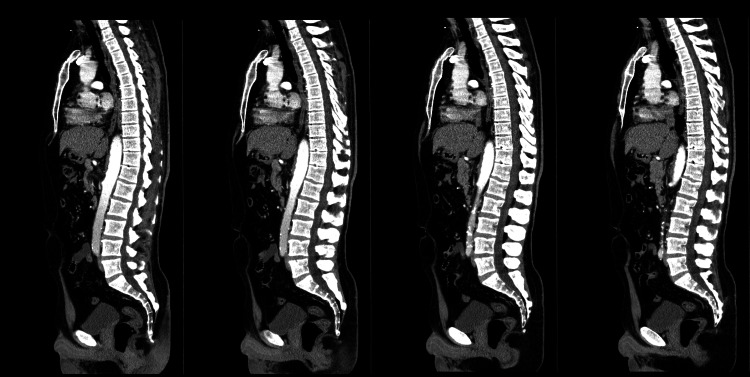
Computed tomography scan at admission in four different sagittal plans.
